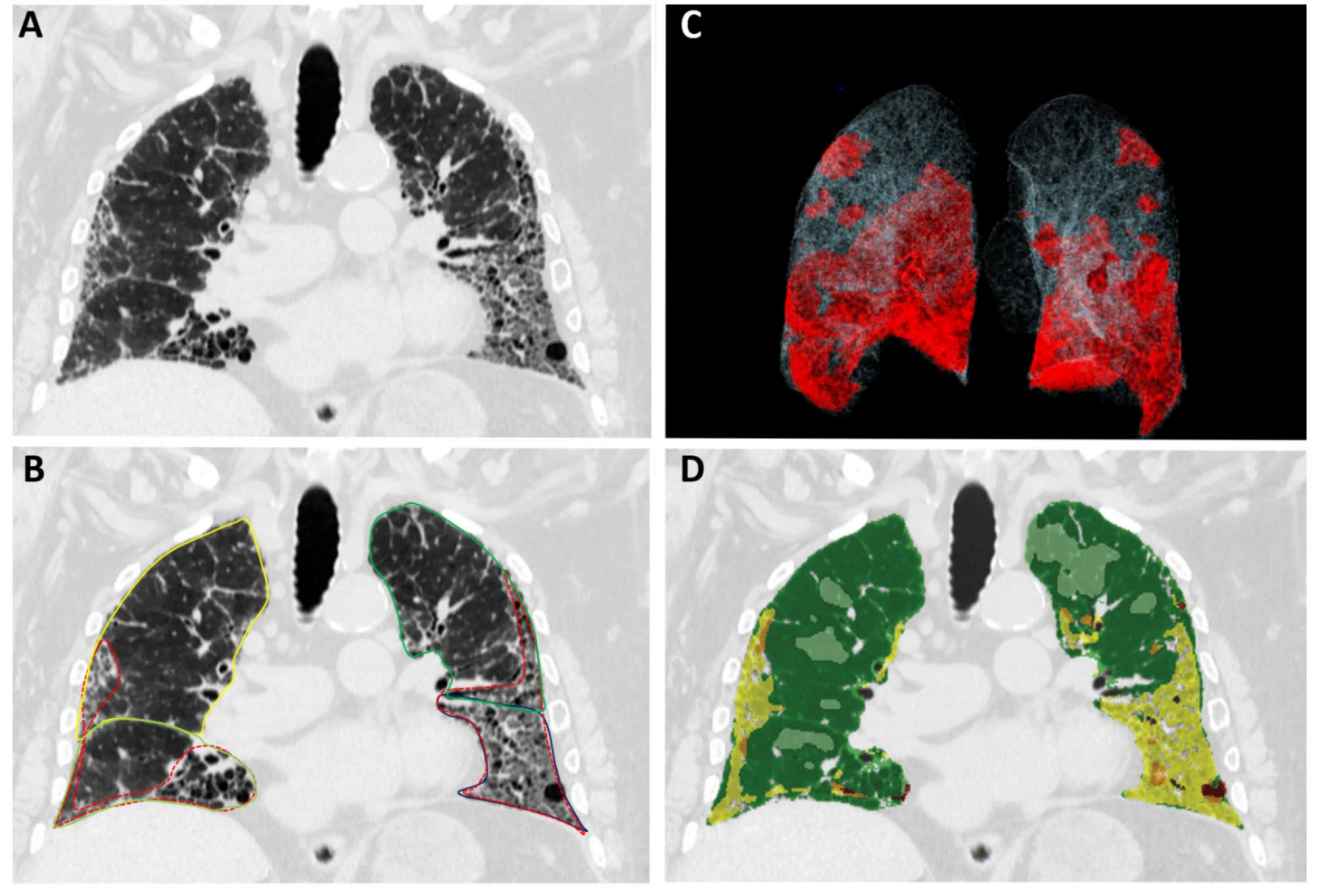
Exemplary results of the multi-texture lung analysis tool and the opacity-based lung analysis tool. CT in coronal view of a 62 year old patient with idiopathic pulmonary fibrosis (A), analyzed by the opacity-based Pneumonia tool (Siemens Healthineers), the red lines represent the segmented fibrotic areas (B) with additional 3D reconstruction (C). (D) Analysis with the multi-texture lung analysis tool (CALIPER, Biomedical imaging resource laboratory, Mayo Clinic) with the yellow areas representing fibrotic altered tissue.
